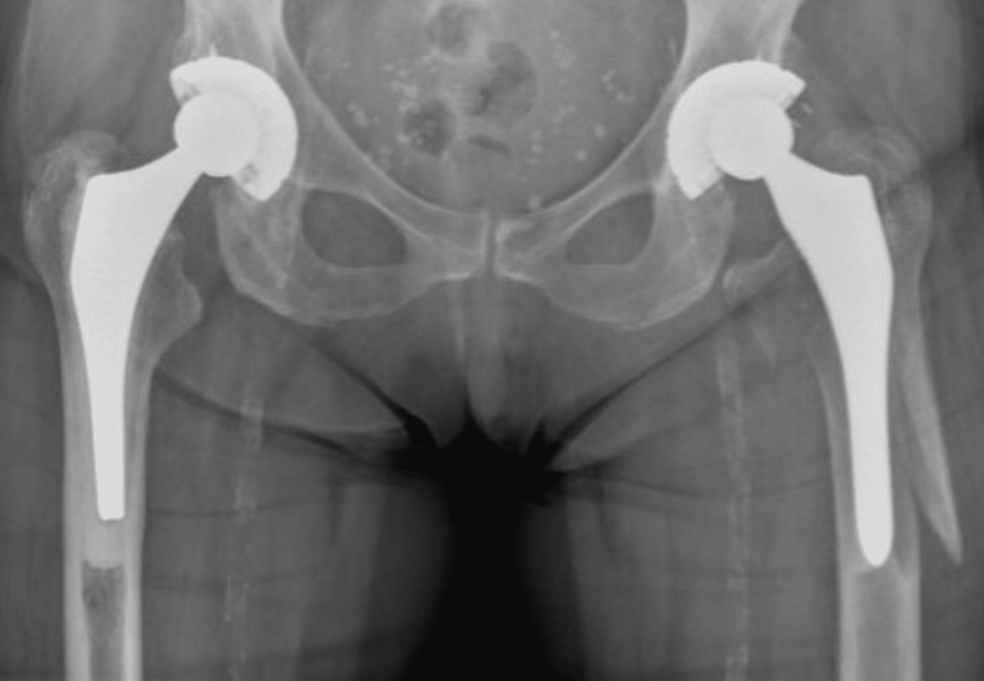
Case C, X-pelvis pre-OR X-ray showing a periprosthetic femoral fracture of the left femur, Vancouver B2 type of fracture
